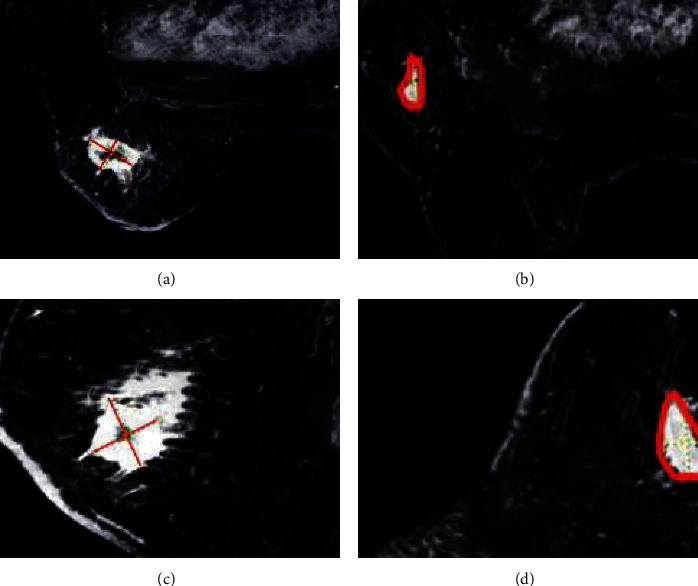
MRI images of the patient before neoadjuvant chemotherapy. (a)-(b) Horizontal images. (c)-(d) Sagittal images. Red areas are breast masses.
